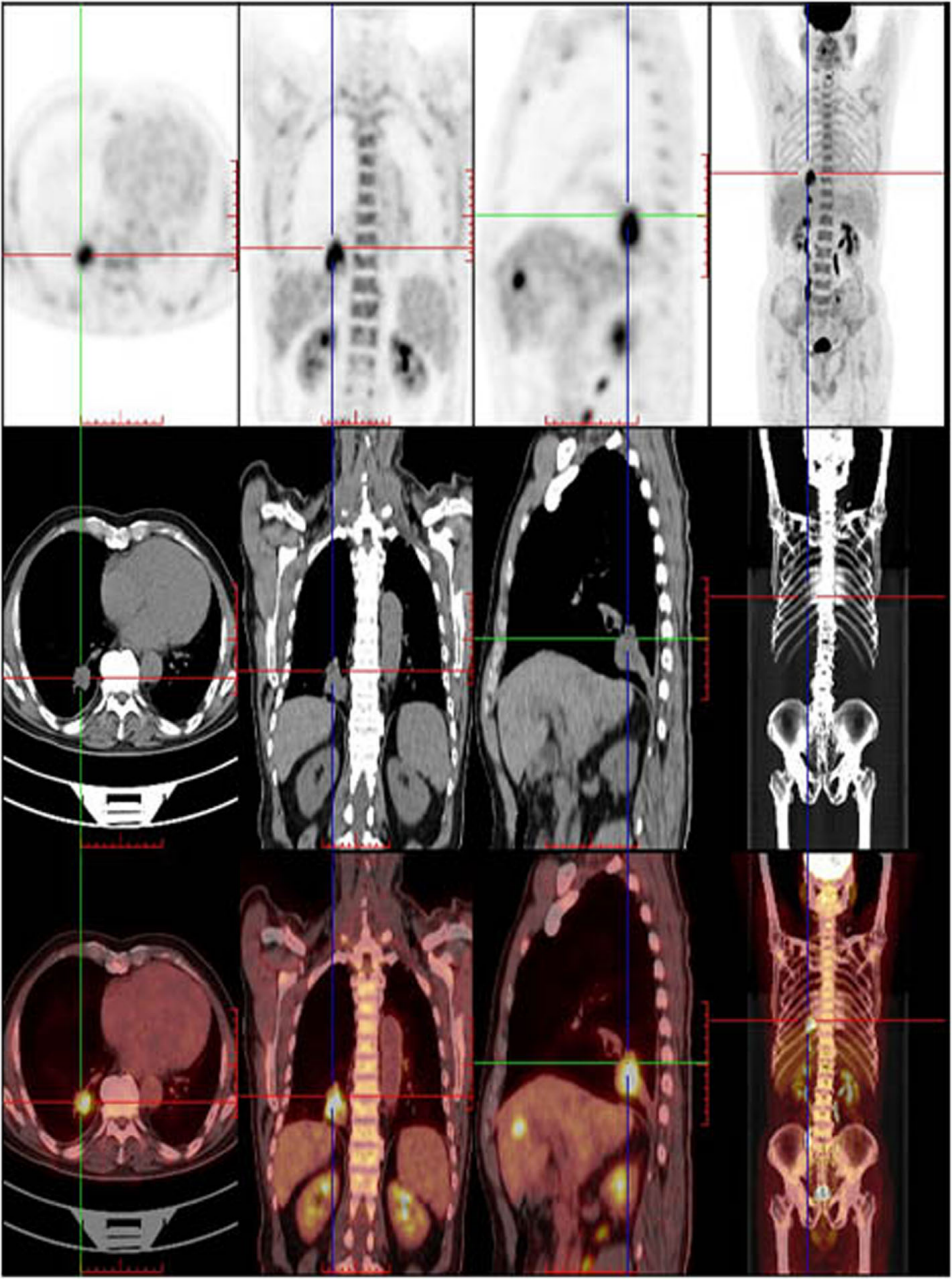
PET-CT revealed a malignant space-occupying lesion in the lower lobe of the right lung. CT, computed tomography; PET, positron emission tomography
